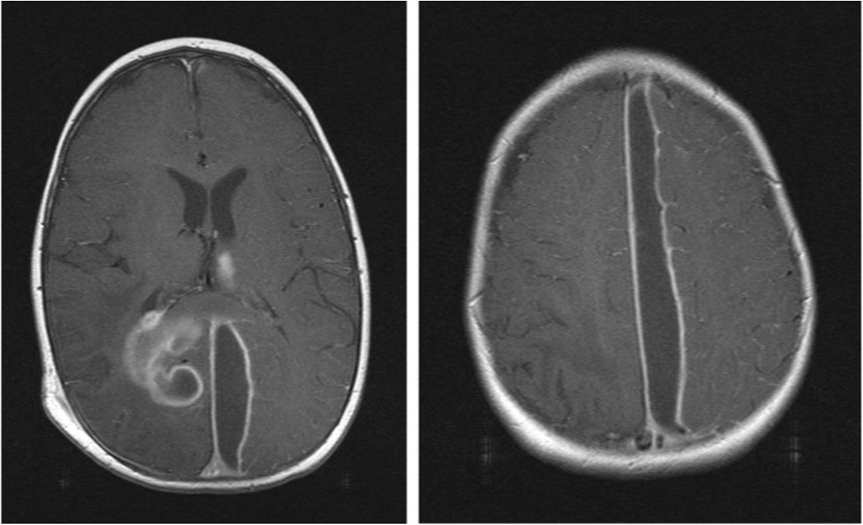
Magnetic resonance imaging 1 week after admission.
